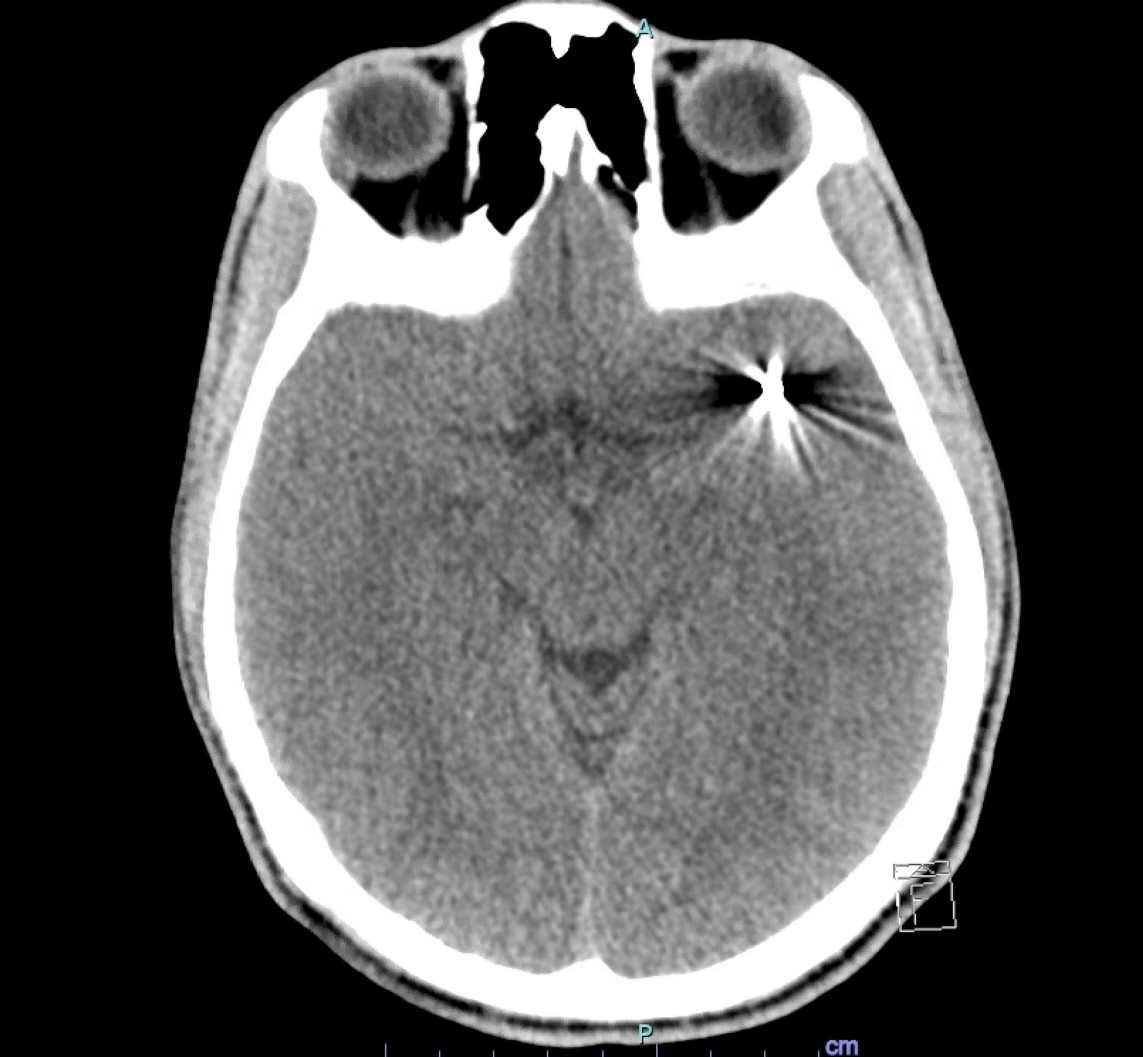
CT head without contrast demonstrating a pellet in the expected location of the left MCA
